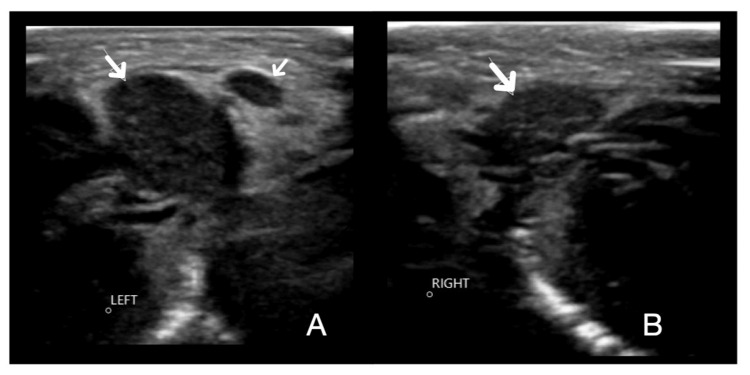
Ultrasound scan of the left and right submandibular glands. (A) Left submandibular gland showing diffuse enlargement (arrow), increased vascularity, and a small local lymph node (arrow) suggestive of inflammation. (B) Right submandibular gland for comparison (arrow).
